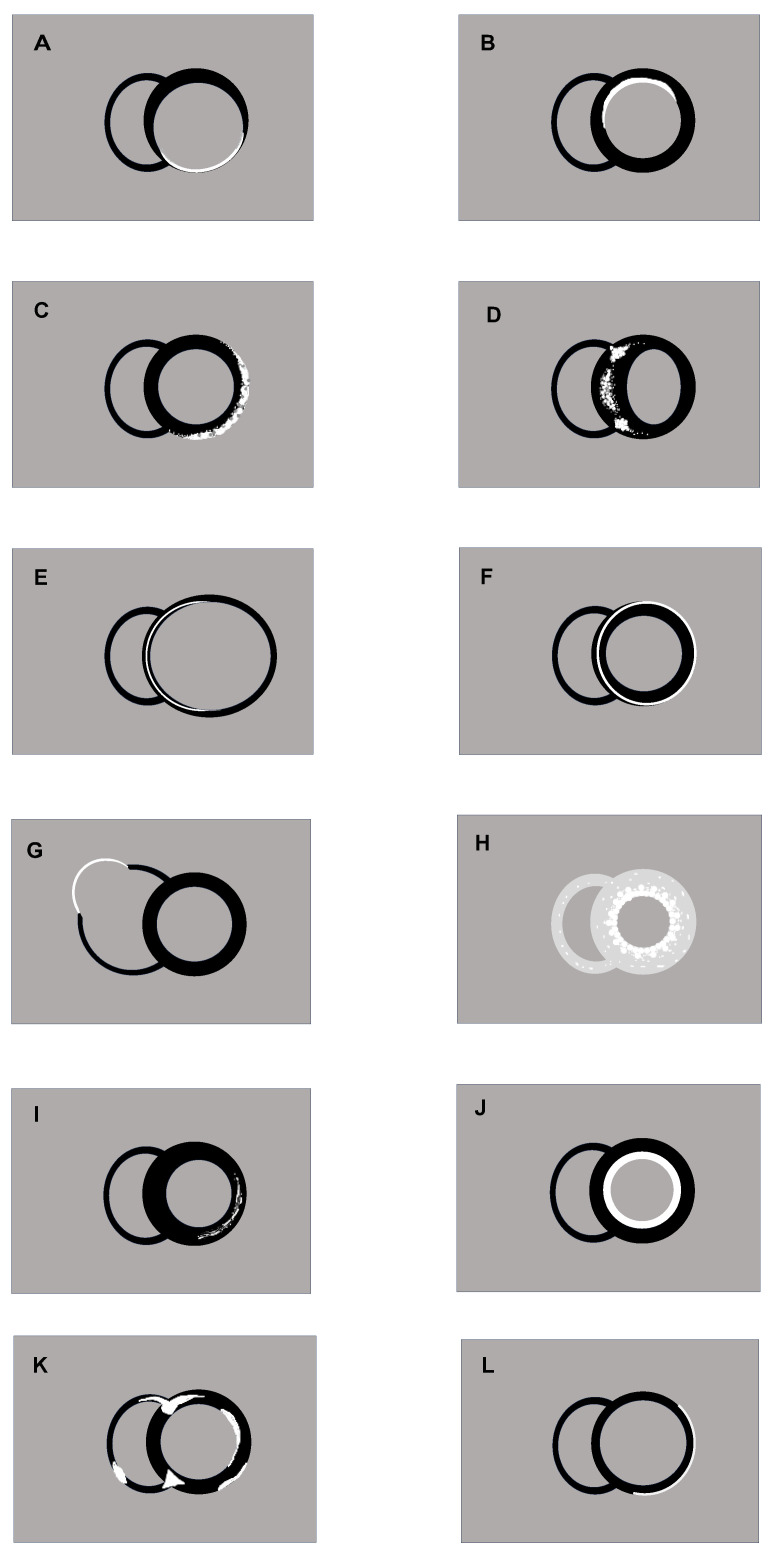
Scheme of typical LGE patterns in short axis view, T1-weighted inversion recovery sequence; black: myocardium without LGE, white: LGE. Please note: the distribution pattern may vary within certain limits and should only be assessed in the context of patient’s history and the whole CMR examination. (A): Transmural ischemic scar with myocardial thinning as consequence of RCA-Infarction. (B): Non-transmural ischemic scar as consequence of LAD-infarction. (C): Myocarditis with subepicardial inferolateral LGE. (D): Classical hypertrophic cardiomyopathy with LGE at the RV insertions and in the area of greatest hypertrophy. (E): Dilated cardiomyopathy with a fine line of intramural septal LGE. (F): Non-dilated cardiomyopathy with ring-like LGE and a septal intramural and lateral epicardial distribution. (G): Classical ARVC with right ventricular dilatation and aneurysms with LGE. (H): Cardiac amyloidosis with strong LGE originating from the subendocardium in the hypertrophied LV and RV myocardium. (I): Anderson–Fabry disease with mild intramural to subepicardial inferolateral LGE and hypertrophy. Note: relatively frequent, unspecific pattern, also possible with increasing pressure load or as post-inflammatory residual. Additional T1 mapping sequences required for differentiation. (J): Endomyocardial fibrosis with LGE in the thickened endocardium, here without thrombosis. (K): Cardiac sarcoidosis with patchy subendocardial, subepicardial or transmural distribution, anterior “Hook-sign” and inferior “Triangle sign” in place of the RV insertions. (L): Cardiac involvement in muscular dystrophy Duchenne with subepicardial lateral LGE in thinned myocardium. Note: also possible in DCM of other origin.
